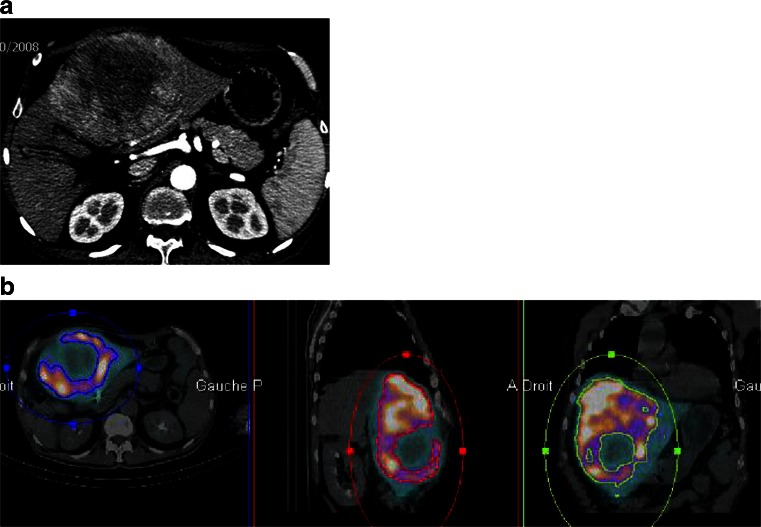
Tumoral VOI delineation using MAA SPECT/CT. a) CT slide: huge tumor of 12.1 × 17.4 × 9.7 cm with a large part of central necrosis. b) MAA/SPECT CT tumor volume segmentation. Based on MAA SPECT/CT, tumor volume is only 900 cc due to a large part of necrosis. Using CT segmentation, tumor volume is 1310 cc. Doing the hypothesis of absence of radioactivity uptake in necrosis, tumor dose based on MAA segmentation (excluding necrosis volume) is 1.45 fold higher than tumor dose based on CT segmentation (including necrosis volume)
